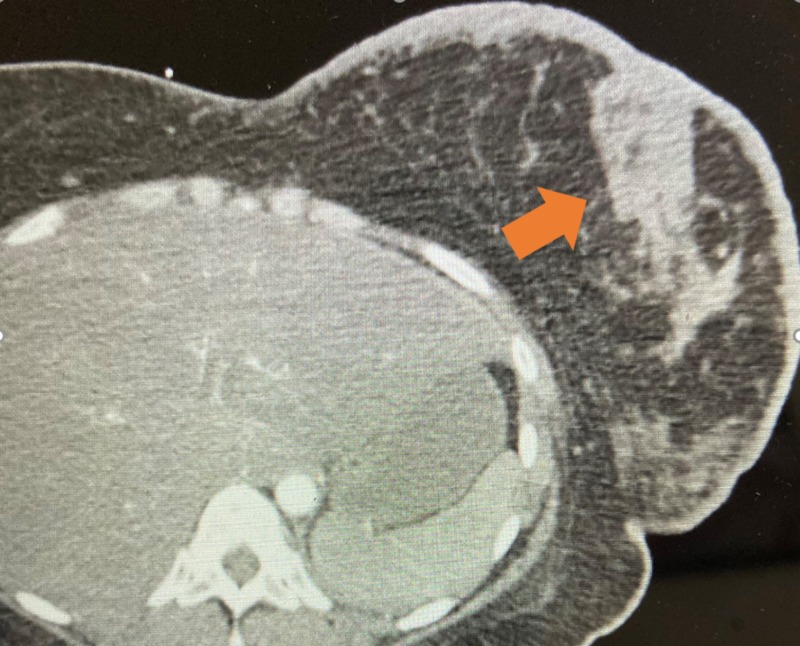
Computed tomography of the chest An abnormal soft tissue density within the left breast was noted (orange arrow), along with additional abnormal skin thickening of the left breast.
